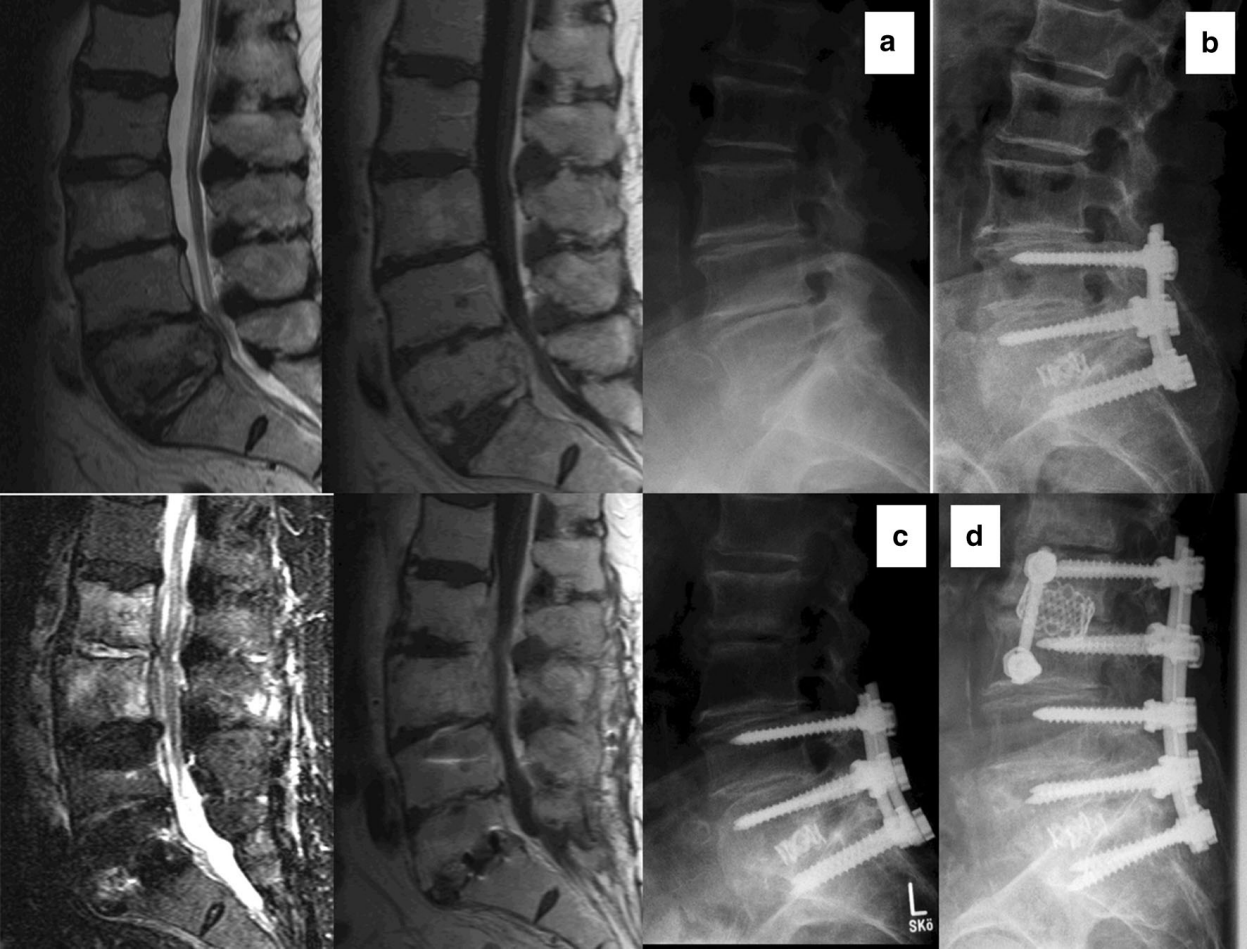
Patient 8: sagittal MRI cuts T2- and T1-weighted, and lateral radiographs; a preoperatively, b after primary operation, c adjacent segment infection, no screw loosening or marked adjacent segment kyphosis, and d last FU after 2.5 years
